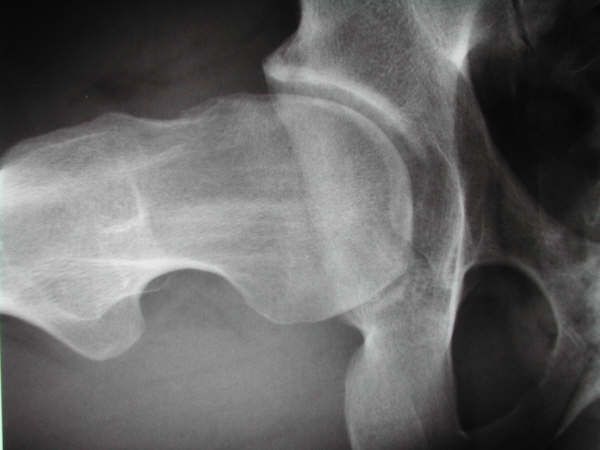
Radiographie de profil du col fémoral montrant une came fémorale chez le patient 1
